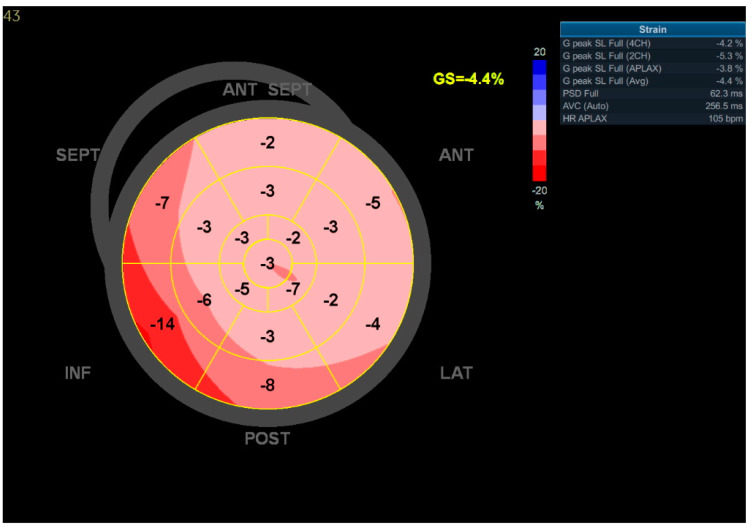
GLS of LV measured by 2D speckle tracking echocardiography in Patient 3. ‘Bull’s-eye’ representation of the regional strains. A global impairment is presented, with a GLS of −4.4%.
